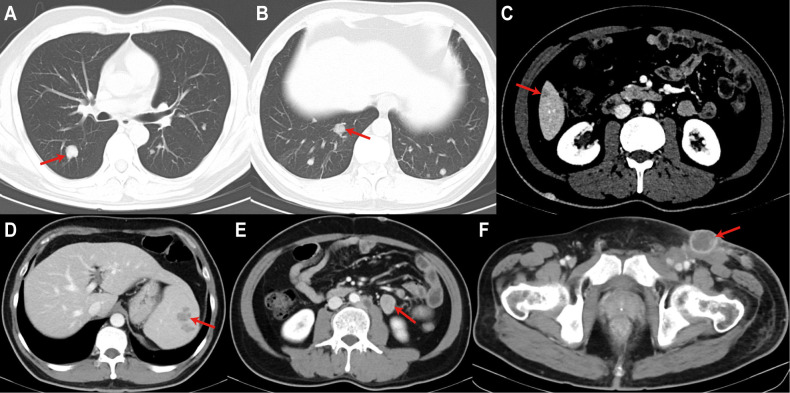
Enhanced CT scan on August 24, 2023, revealed multiple systemic organ metastases. (A, B) Multiple nodules in both lungs; (C) A subcapsular nodule in the inferior segment of the right anterior hepatic lobe; (D) Multiple nodules in the spleen; (E) A nodule in the left adrenal gland; (F) Enlarged lymph nodes in the left inguinal region. All of the above findings are considered metastatic.
